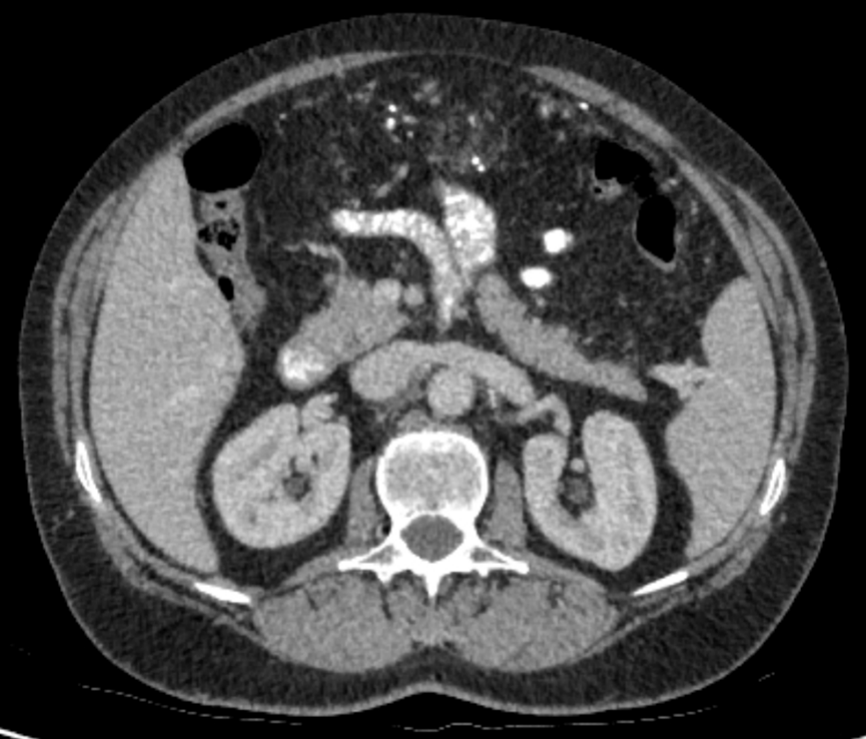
CT axial slice of upper/lower abdomen showing thickening of the omentum which contains a mantle of speckled calcifications.
